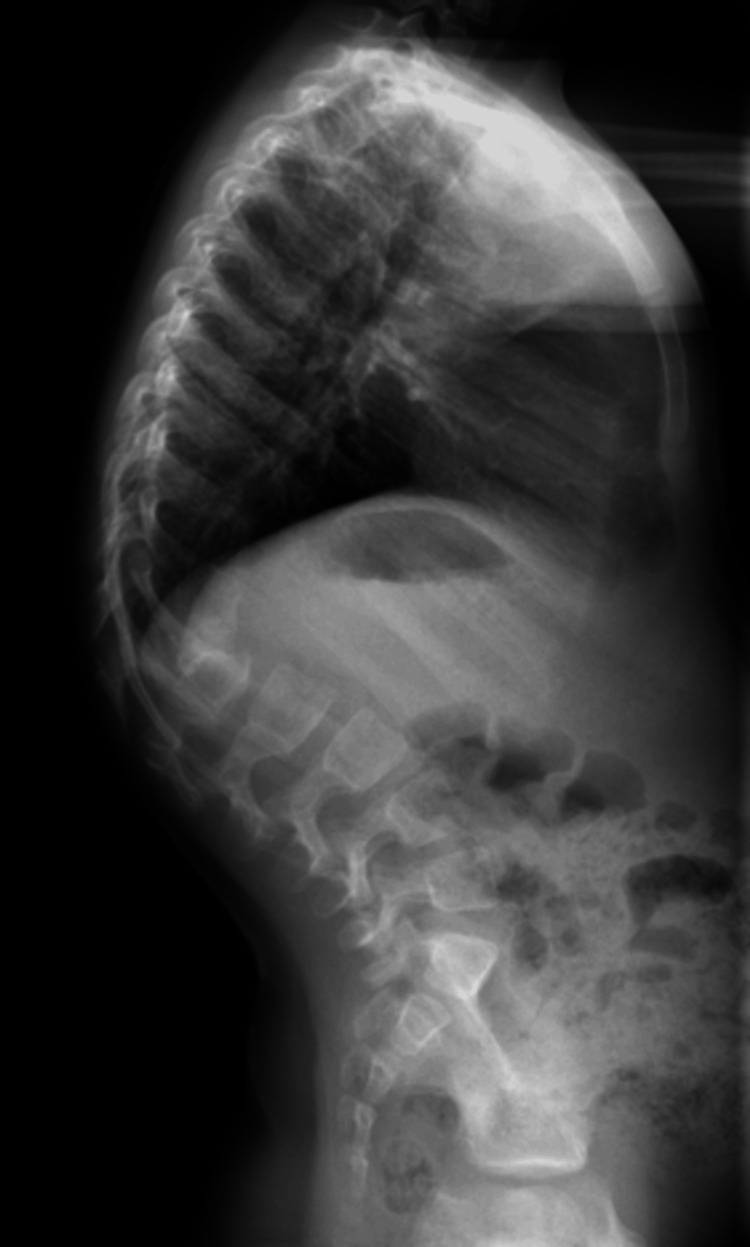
A pre-operative lateral radiograph of the thoracolumbar spine depicts the 70-degree angle of congenital kyphosis and the two hemivertebrae of T10 and T12.
